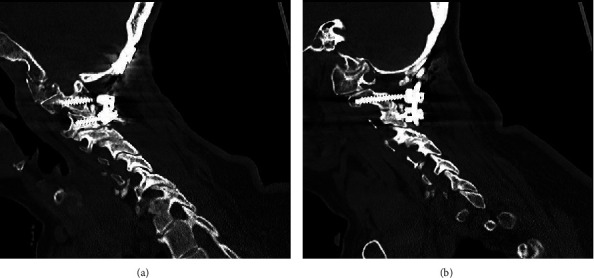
(a, b) Cervical CT one year after surgery demonstrating the occipital-C3 construct.
